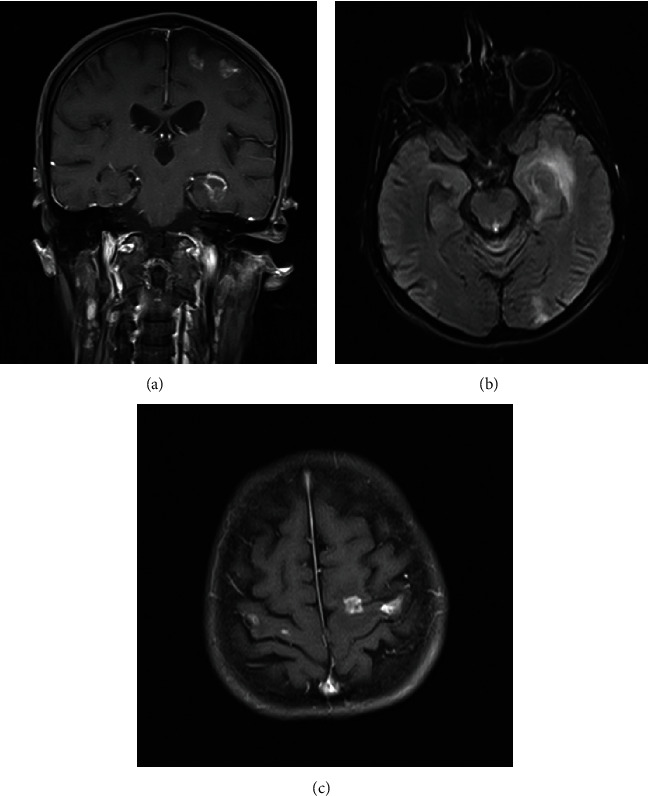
Magnetic resonance imaging (MRI): T1 with contrast in coronal (a) and axial planes (b, c): multiple ring-enhancing lesions in the left and right cerebral cortices.
